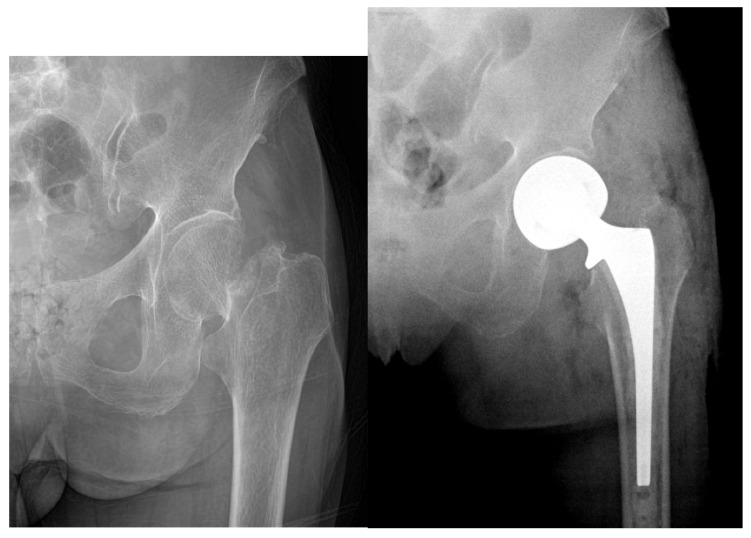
Example radiographs demonstrating uncemented hemiarthroplasty Garden III fracture (Left: Preoperative, right: Postoperative).
